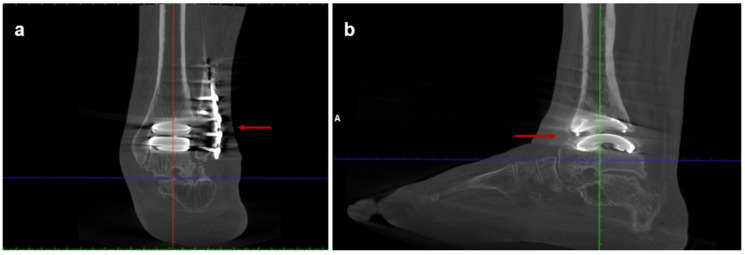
Postoperative coronal (a) and sagittal (b) WBCT views at 3-year follow-up of Case two. The arrow in panel (a) indicates restoration of alignment, while the arrow in panel (b) indicates absence of radiographic signs of loosening.
